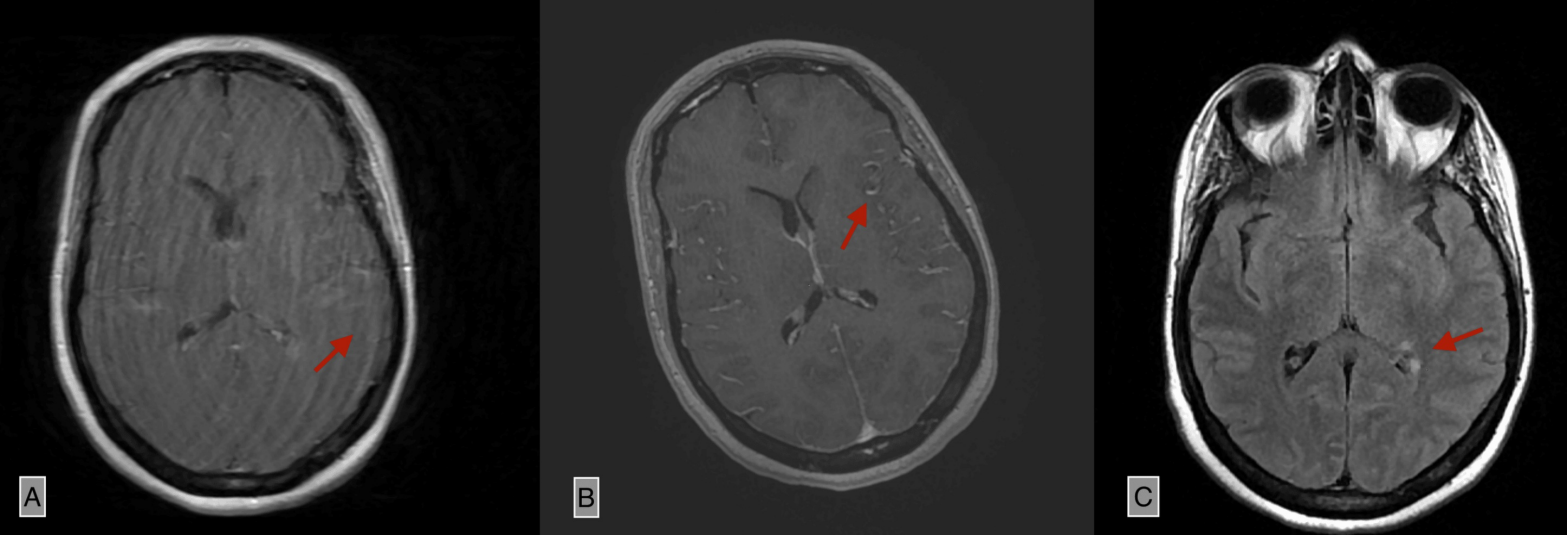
Contrast-enhanced MRI showing diffuse supratentorial leptomeningeal thickening and hyperintense signals in the left periventricular white matter, with post-contrast enhancement. These findings are suggestive of lupus-related vascular involvement with leptomeningitis (A) Axial T1-weighted post-contrast sequence showing periventricular contrast enhancement (arrow). (B) Axial T1-weighted post-contrast sequence demonstrating diffuse supratentorial leptomeningeal enhancement (arrow). (C) Axial FLAIR sequence showing periventricular hyperintense signal (arrow).
